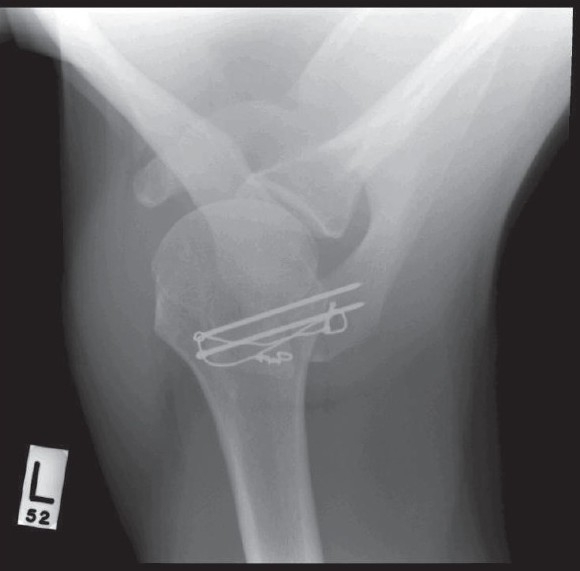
Postoperative x-ray demonstrating the tension band wires holding the fixation
